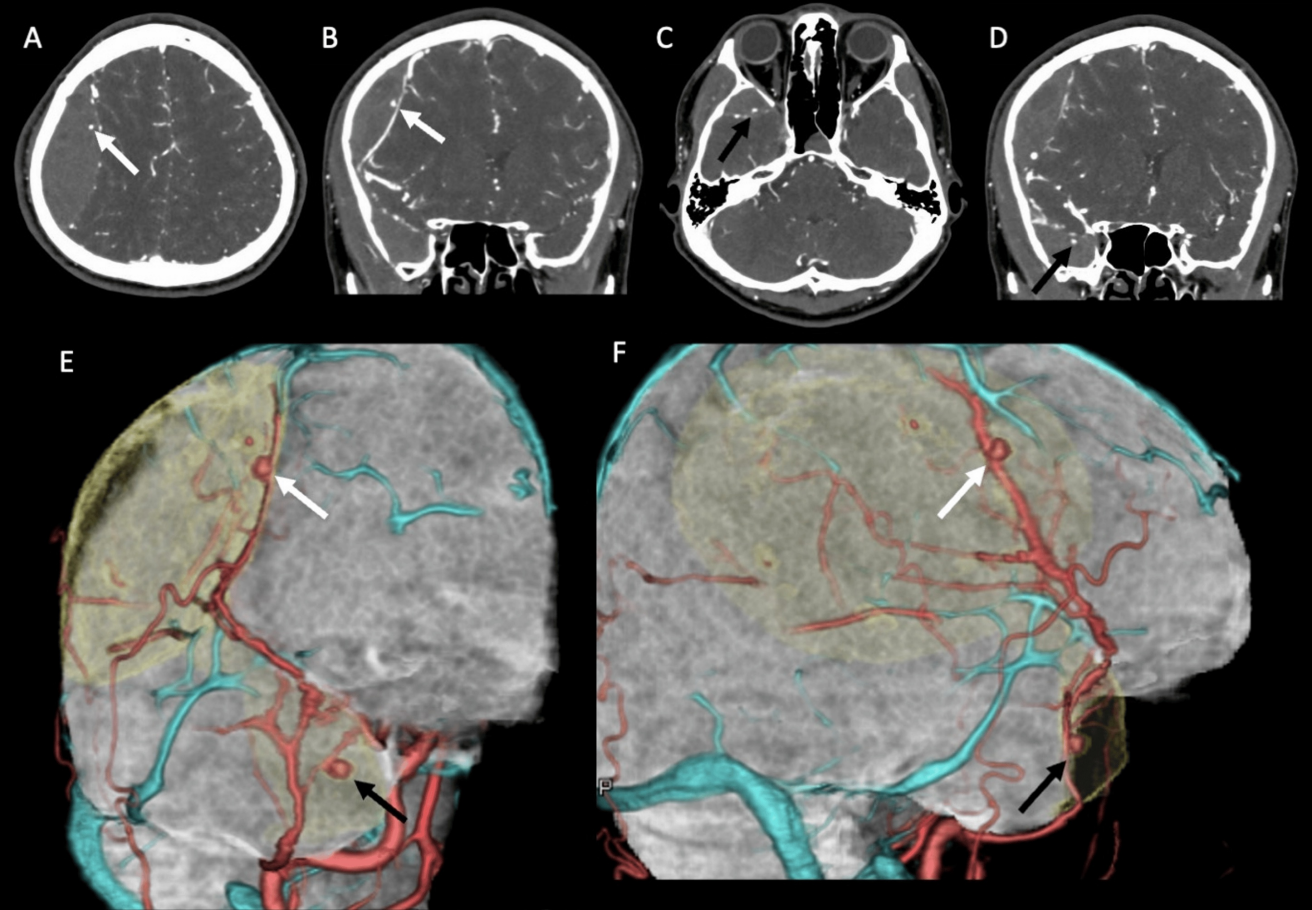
Preoperative contrast-enhanced CT image and CT angiography (A-B) Contrast-enhanced axial (A) and coronal (B) CT scans demonstrated the contrast-medium extravasation (white arrow) in the small AEDH at the right temporoparietal region. (C-D) Contrast-enhanced axial (C) and coronal (D) CT scans demonstrated the contrast-medium extravasation (black arrow) in the small AEDH at the right-middle cranial fossa. (E-F) An anterior view (E) and a right lateral view (F) of CT angiographies demonstrated two pseudoaneurysms in the right temporoparietal AEDH (white arrow) and right middle-cranial fossa AEDH (black arrow) along with the right middle meningeal artery. The AEDHs are colored in light yellow. AEDH: acute epidural hematoma; CT: computed tomography
